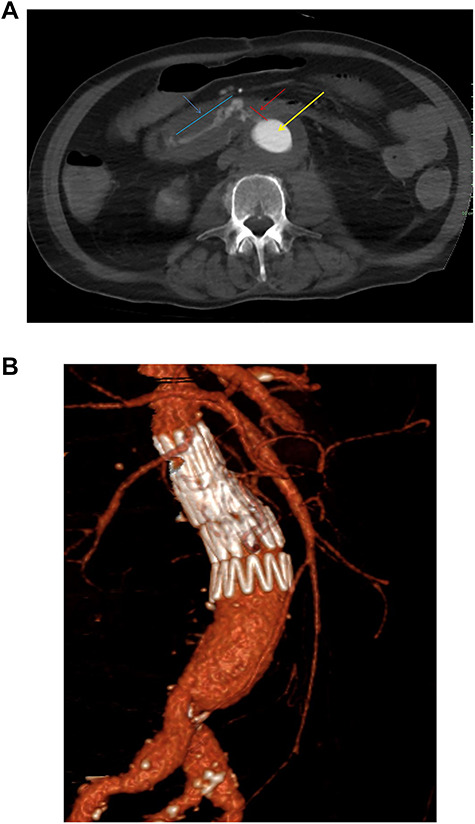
(a) Axial thick slice of a CT with IV contrast in the arterial phase shows the lumen of the surgical graft (yellow arrow), clear contrast opacification within the fistula (red line and arrow) and the duodenum (blue line and arrow) consistent with active haemorrhage; (b) sagittal reconstruction of a CT with IV contrast in the arterial phase showing the overlapping aortic stent grafts inserted to exclude the AEF from the circulation.
